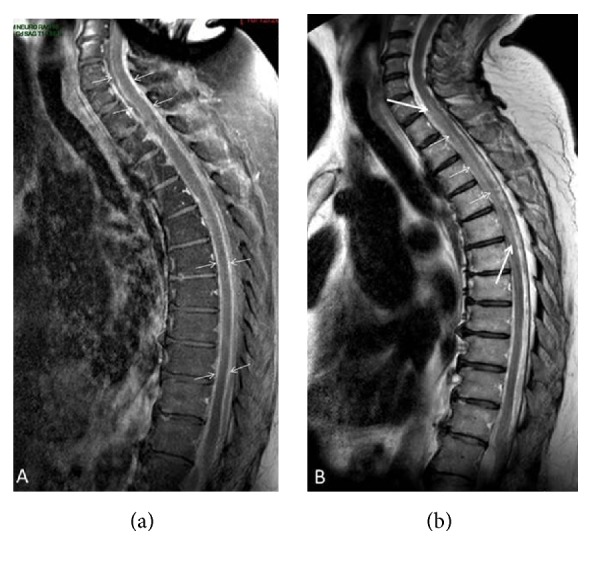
Follow-up MR examination of the cervicodorsal segments. (a) Midsagittal CE T1-weighted view showing strong enhancement of both dura and pia (between arrows) surrounding spinal cord. (b) Midsagittal T2-weighted view showing relative hypersignal intensity within upper anterior spinal artery territory between T1/T2 and T5/T6 disks (arrows) corresponding to spinal cord infarction.
